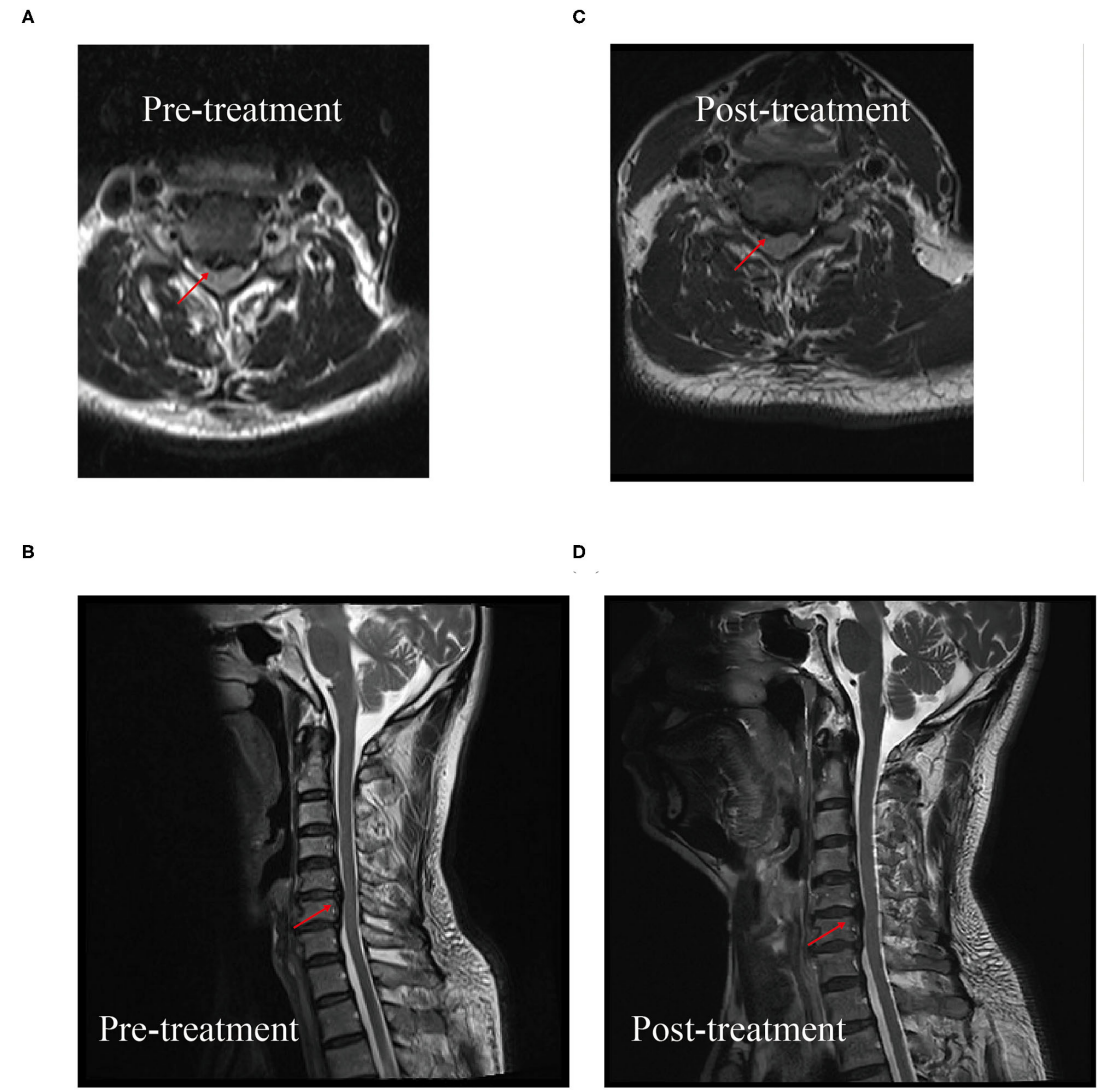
Comparison of MRI between pre- (A,B) and post-treatment (C,D) in a male patient who underwent coblation surgery. The red arrow indicates the cervical disc herniation in sagittal plane.
